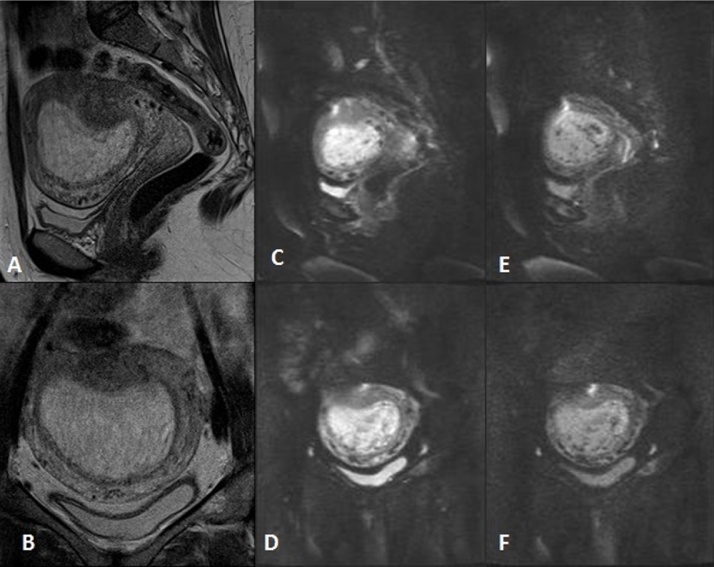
A 30-year-old woman with a molar pregnancy. (A) Sagittal and (B) perpendicular to the endometrial line, T2-weighted MR images show a snowstorm appearance and focal, low-signal myometrial thickening due to contraction. The endometrial line is sharply preserved (C, E) sagittally and (D, F) perpendicular to the endometrial line on DWI with b values of 800 and 400, respectively, and shows prominent myometrial vascularity.
